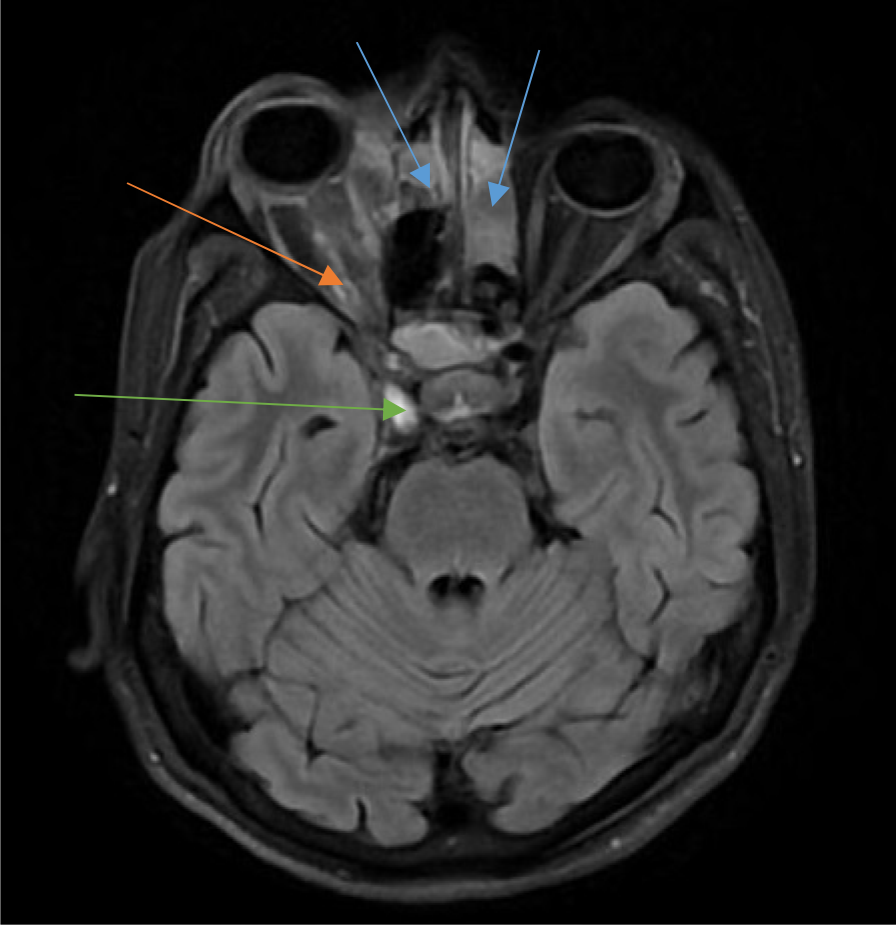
Axial section of contrast-enhanced T1-weighted image of an MRI of the brain in a patient with rhino-orbitocerebral mucormycosis showing heterogeneously enhancing mucosal thickening in bilateral ethmoid sinuses (blue arrows) with enhancing soft tissue surrounding the intra-orbital segment of the right optic nerve (red arrow). Also noted is lack of flow void in the right cavernous sinus indicating thrombosis (green arrow)
